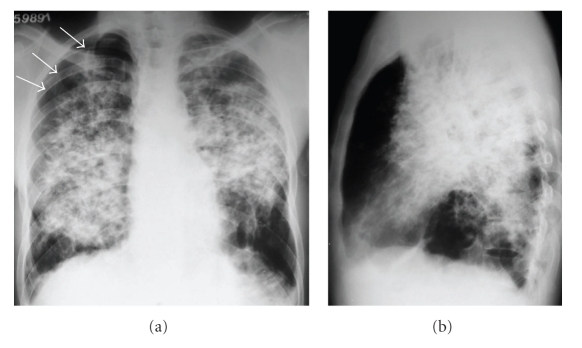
Chest radiographs in anteroposterior (a) and lateral (b) incidences showing a small right pneumothorax (arrows), and bilateral nonhomogeneous opacities, predominantly in the middle third of both lungs, with posterior predominance. Emphysematous areas are seen in the lung bases.
